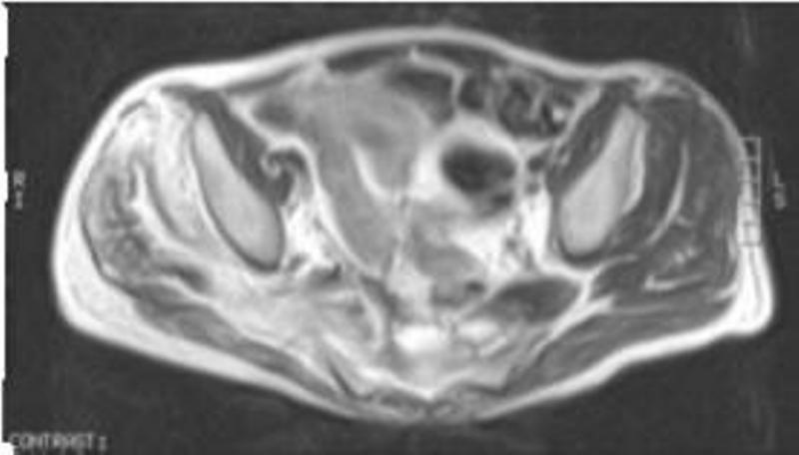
CT showing gas in the bladder and two locules of gas in the soft tissue just outside piriformis muscle and adjacent to the right femur.
